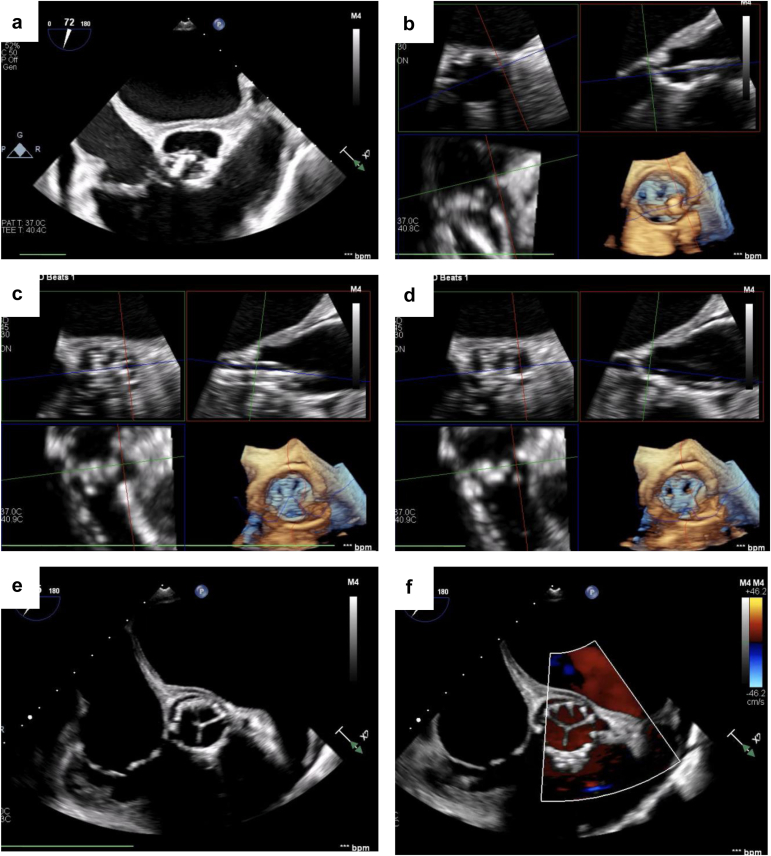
Representative transesophageal echocardiographic imaging of UNICORN-assisted TAVR deployment in a native aortic valve. (a) Mid-esophageal aortic valve short axis demonstrating calcified aortic valve. (b) Leaflet modification using the UNICORN technique. (c) Deployment of the transcatheter heart valve. (d-f) Final position of the implanted TAVR valve. Abbreviations: TAVR, transcatheter aortic valve replacement; UNICORN, Undermining Iatrogenic Coronary Obstruction with Radiofrequency Needle.
